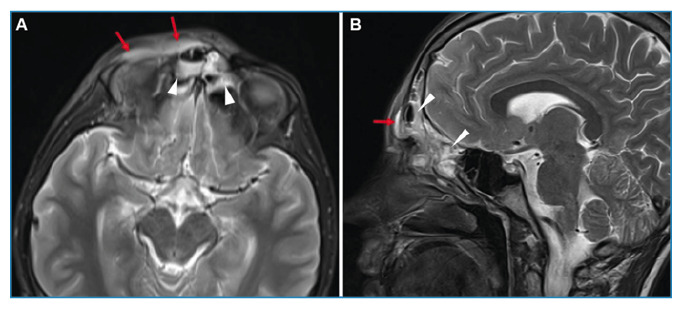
Axial (A) and sagittal (B) section T2-weighted brain MRI show diffuse intensity increases (arrowheads) of sinusitis in the ethmoid cells and frontal sinus. Additionally, greater intensity and thickness (red arrows) are observed in the right periorbital area.
